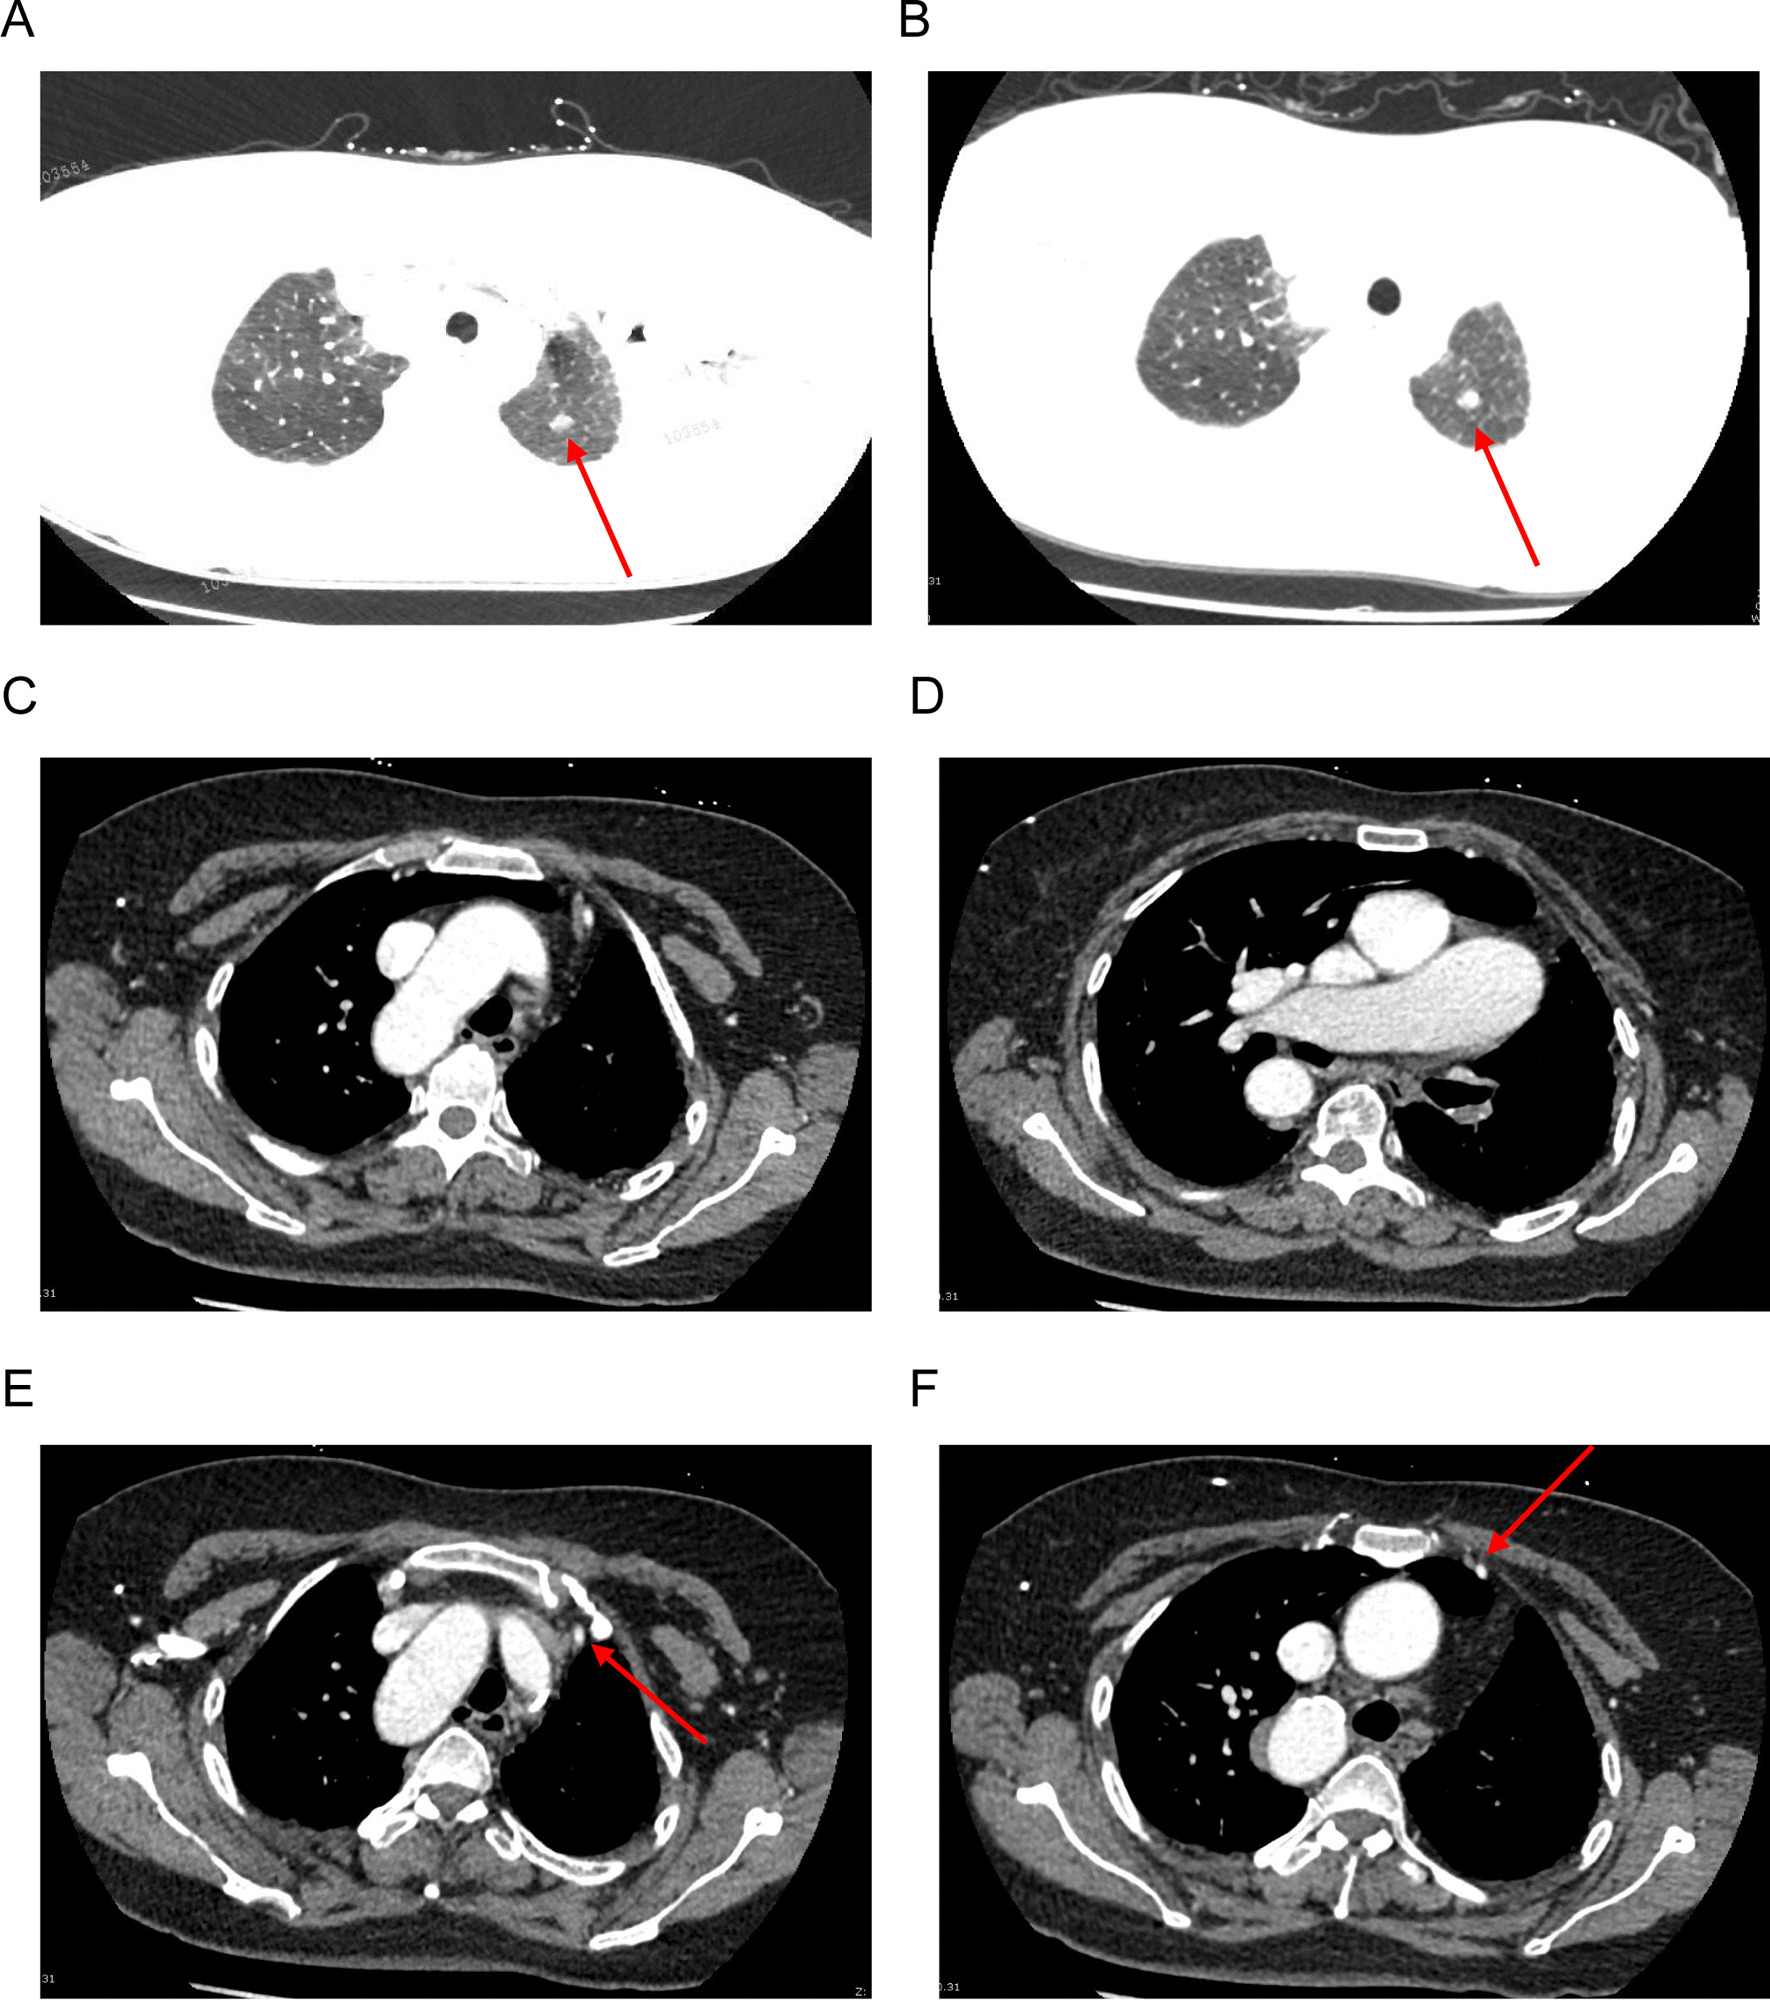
A year earlier (A) and pre-operative (B) enhanced chest computed CT of mixed ground glass opacity in the upper lobe of the left lung (C) a right aortic arch, D absence of the left pulmonary artery, E, F the internal thoracic artery extending to the left lung
